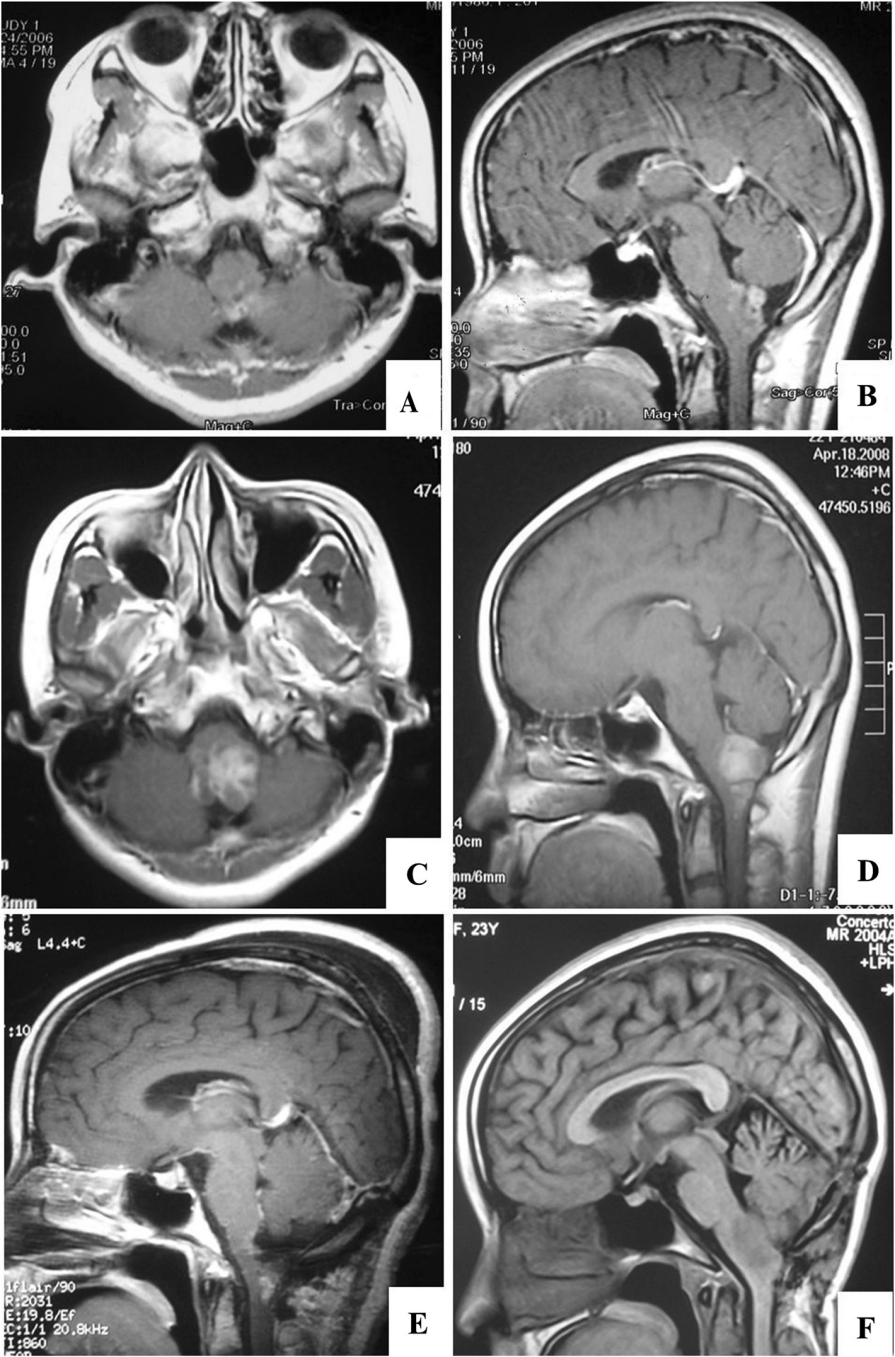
Brain magnetic resonance (MRI) scans of the case. Axonal (A) and sagittal (B) T1-weighted brain MRI 5 years before gamma knife treatment, depicting an enhancing lesion in the dorsal medulla oblongata. Preoperative MRI scans showing growth of the tumor (C, D). Post-operative brain MRI (10 days after surgery) demonstrating complete resection of the lesion (E). MRI scans 7 months after surgery, demonstrating recurrence of the tumor (F).
